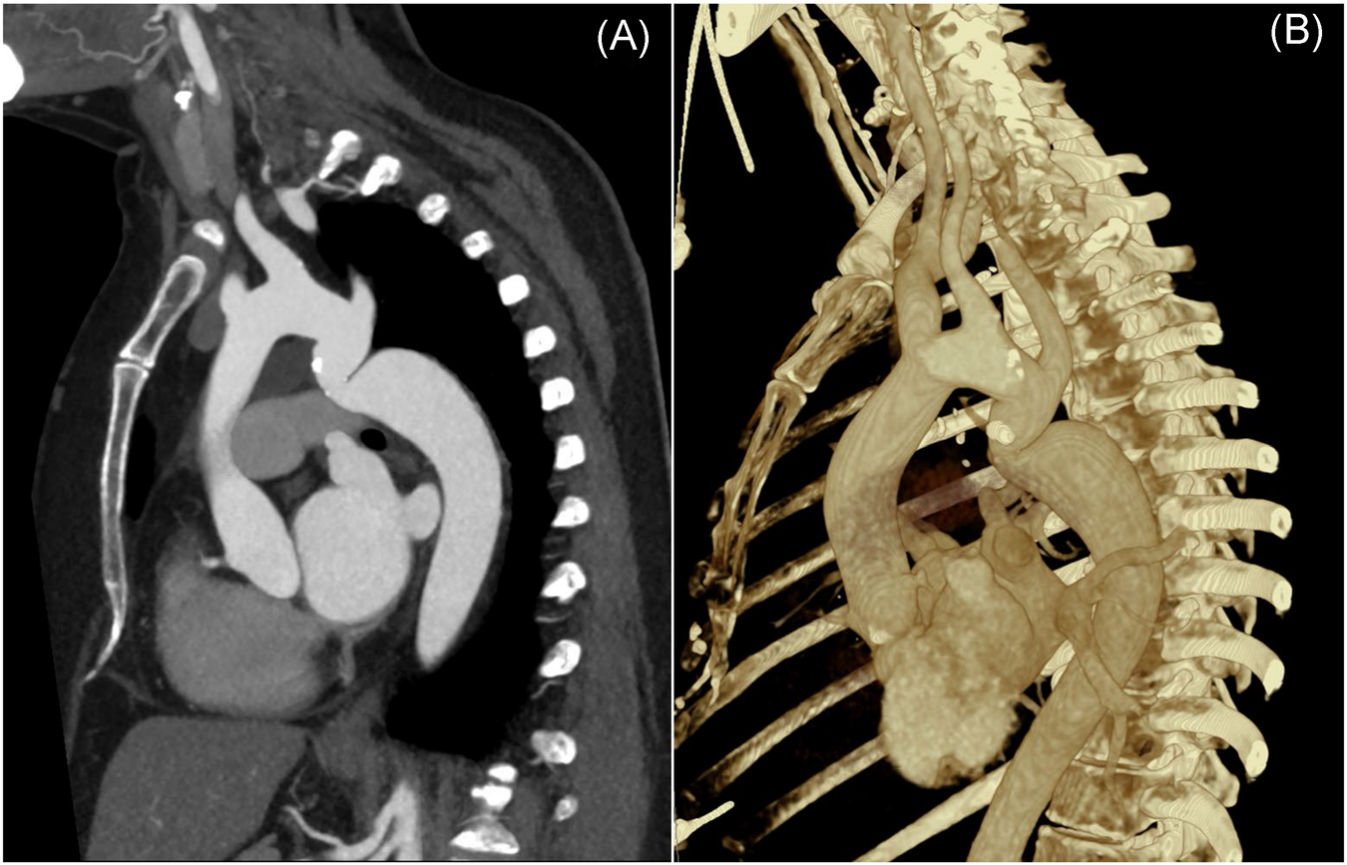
Preoperative computed tomography (CT) in sagittal axis showing aortic coarctation (A) native view (B) CT‐based three‐dimensional reconstruction
